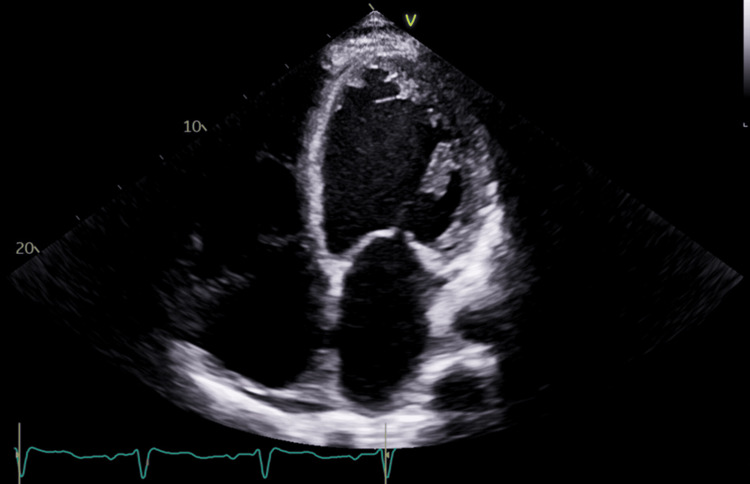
Apical four-chamber view echocardiogram showing biventricular enlargement with severely reduced systolic function (EF 15%). EF: ejection fraction
